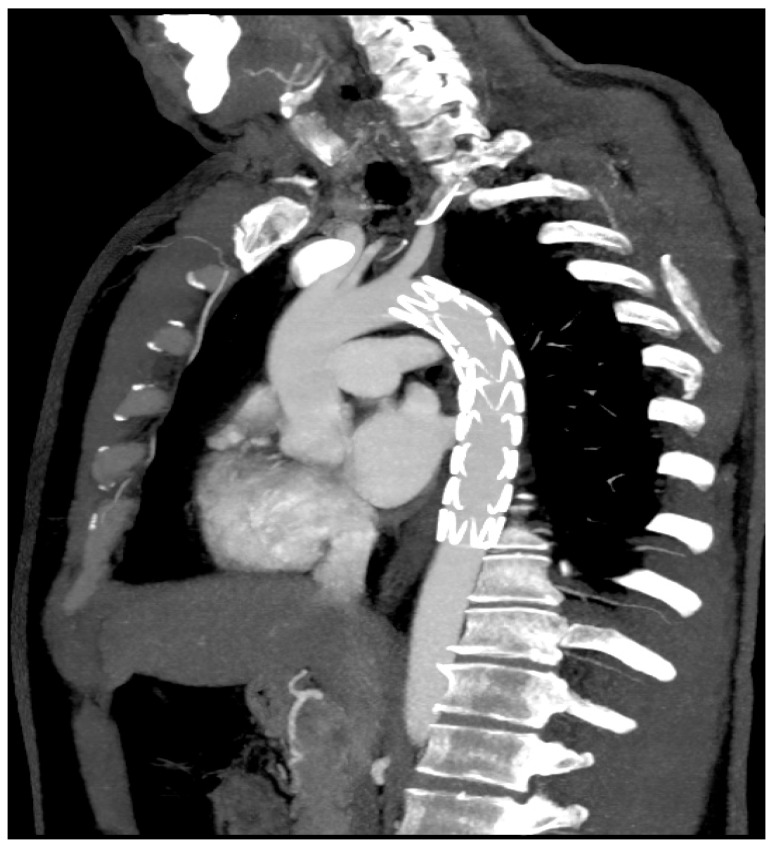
Chest CTA at 1 month after discharge.
